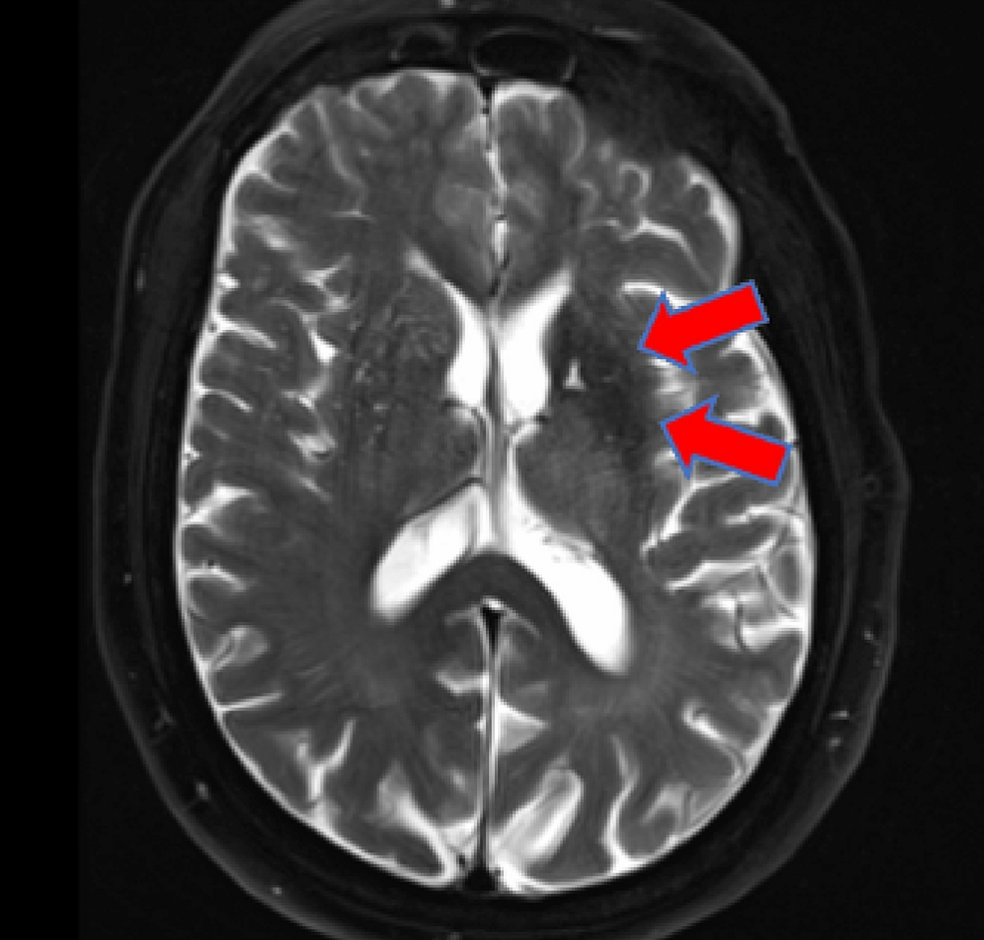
T2-weighted FLAIR MRI Hypointensity in the left lentiform nucleus (arrows) FLAIR: fluid-attenuated inversion recovery, MRI: magnetic resonance imaging
